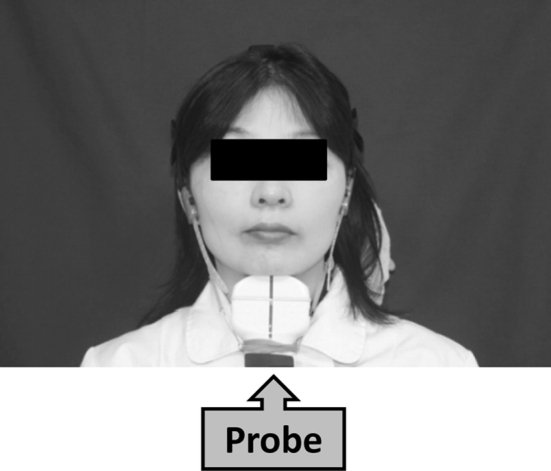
Position of ultrasonic probe in frontal view
